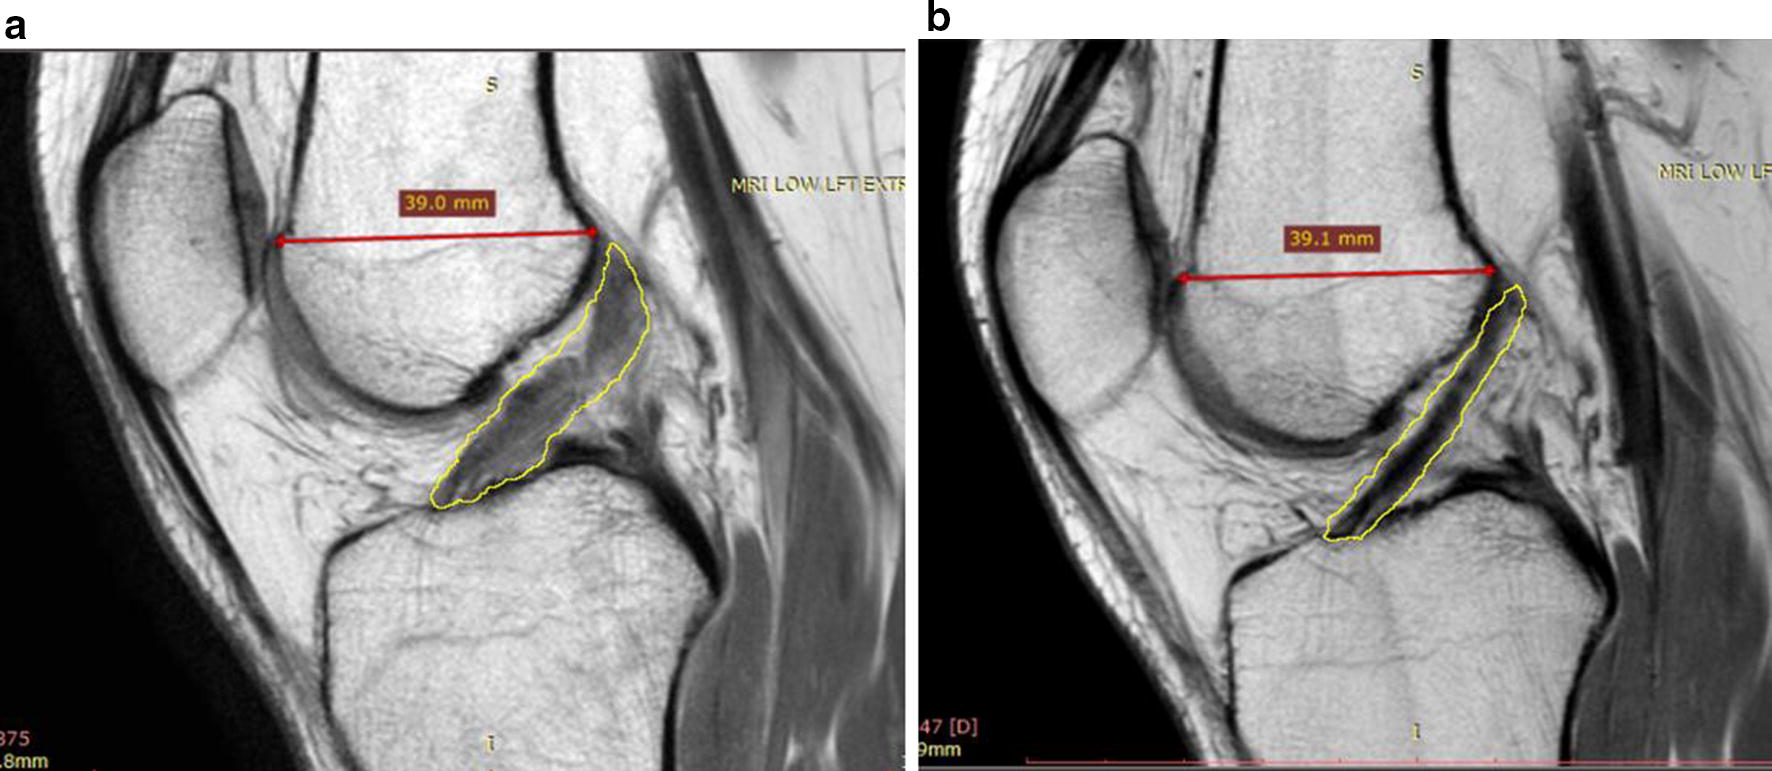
MRIs with ACL outlined using ImageJ software showing progression of ACL healing after BMC treatment. a Pre-treatment MRI showing injured ACL. b MRI at 22 months post-treatment displaying characteristics typical of an uninjured ACL (darker, more dense) indicating healing
